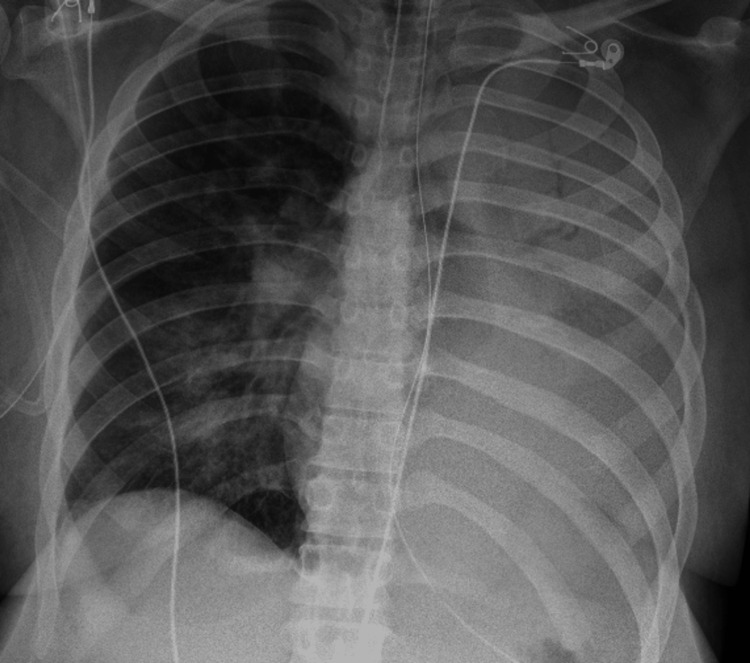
Near-complete white-out of one hemithorax Chest radiograph demonstrating complete opacification of the left hemithorax with mediastinal shift toward the left, consistent with collapse of the left lung secondary to airway obstruction
